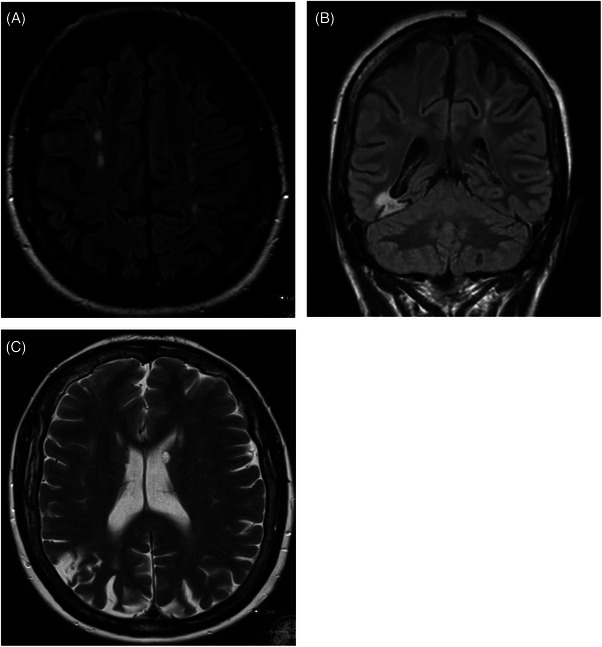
Serial cerebral magnetic resonance imaging of a patient with antiphospholipid syndrome over a 10‐year period. (A) Initial axial fluid attenuated recovery sequence (FLAIR) sequence demonstrating deep watershed infarcts (B) coronal FLAIR sequence acquired 5 years after the initial scan, demonstrating a right occipitotemporal (posterior cerebral artery) territory embolic infarct. (C) Axial T2 sequence acquired 10‐years after the initial scan, demonstrating an external watershed infarct interposed between the middle and posterior cerebral arterial territories.
